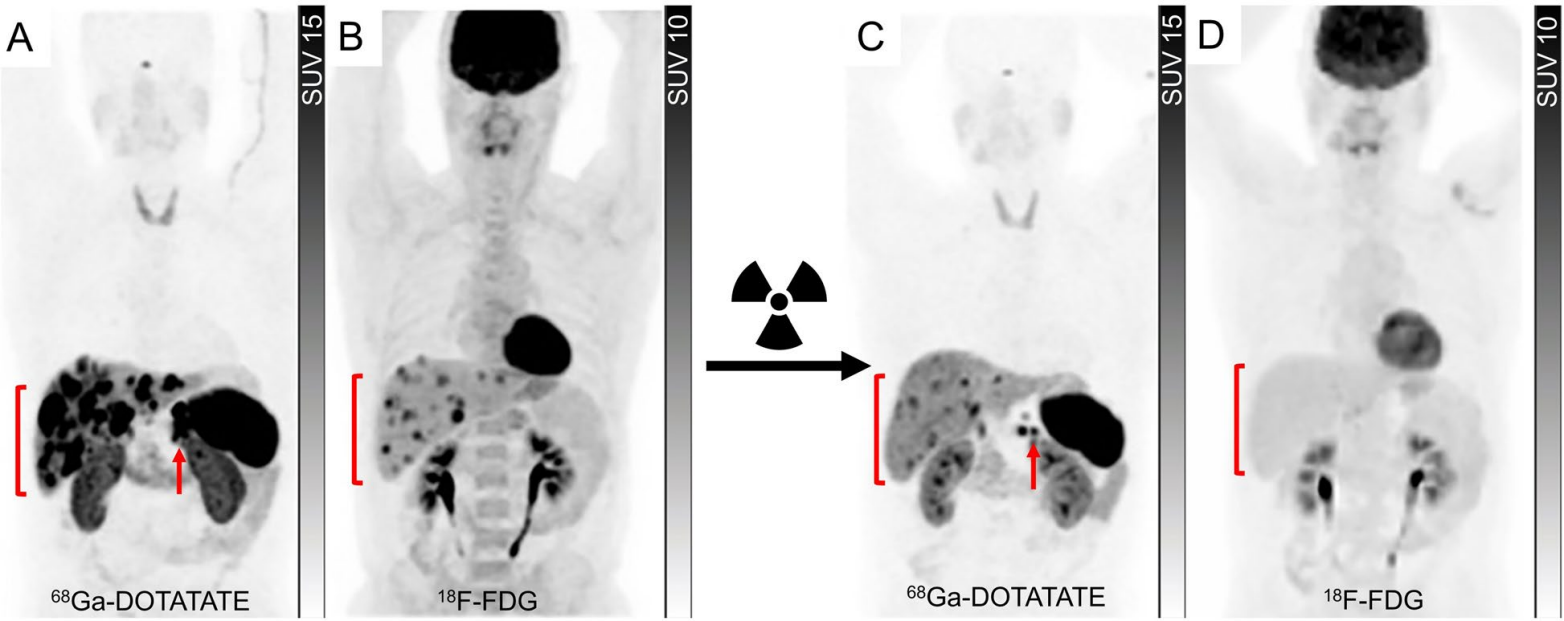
Monitoring of PRRT using dual tracer approach. A patient with pancreatic NET (G3, Ki-67 30%) with ^68^Ga-DOTATATE PET (A) showed a large volume of intensely tracer-avid hepatic metastases (bracket) and a pancreatic mass (arrow). Contemporaneous ^18^F-FDG PET (B) showed some of the hepatic lesions are moderate to intensely avid (bracket) but with no ^18^F-FDG-avid/non-^68^Ga-DOTATATE-avid disease; hence the patient was deemed suitable for PRRT. After 4 cycles of ^177^Lu-DOTATATE, There was a significant reduction in the tumour burden on ^68^Ga-DOTATATE PET (C, bracket and arrow) and a complete metabolic response on ^18^F-FDG PET (D, bracket). Despite persisting SSTR-expressing disease, the loss of ^18^F-FDG avidity suggested an improved prognosis. The patient remained well more than 10-years after initial PRRT despite requiring additional PRRT for relapsing SSTR-expressing disease
